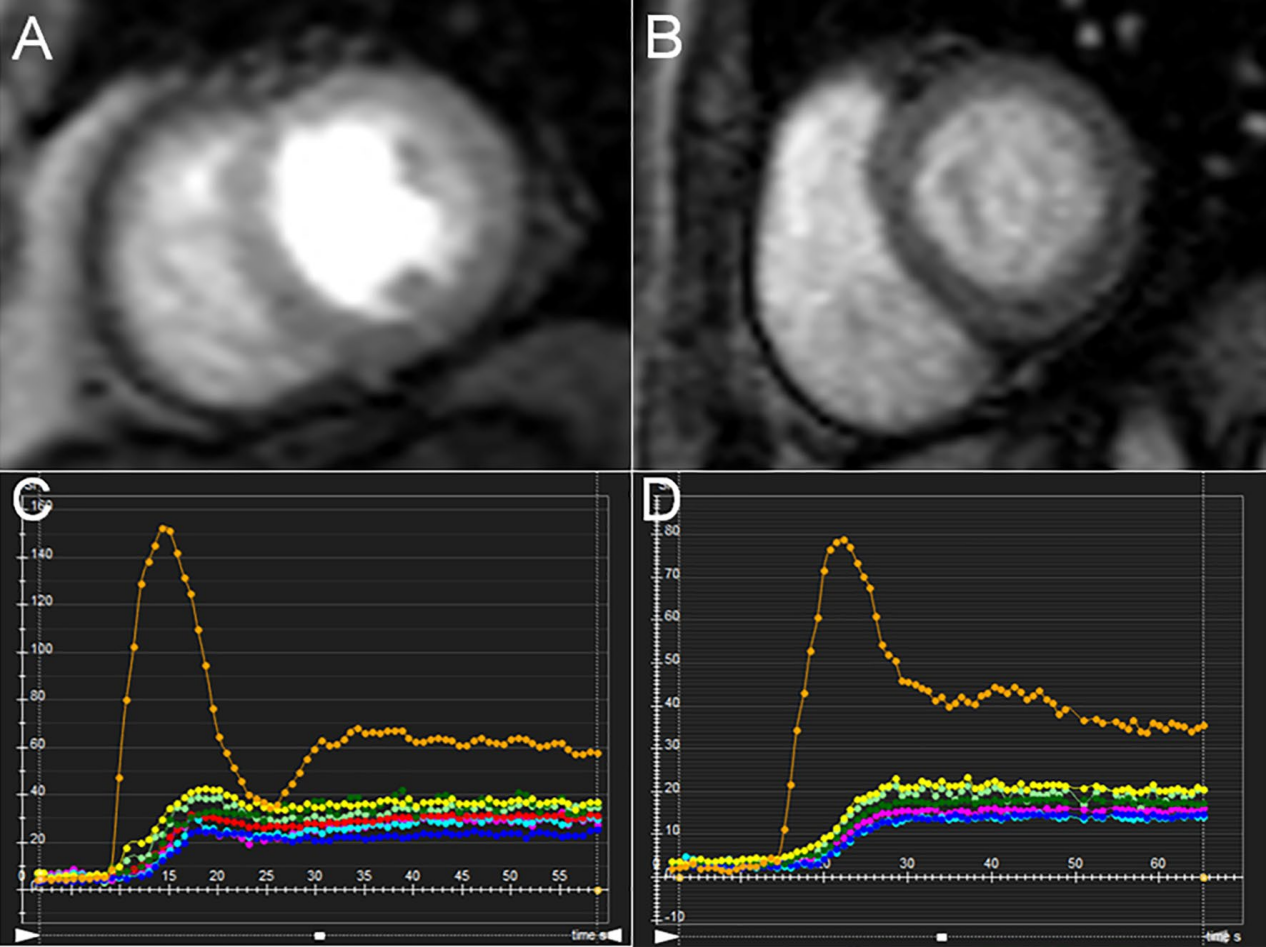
Representative first-pass myocardial perfusion MR images and signal intensity-time curves in normal controls (A, C) and patients with T2DM (B, D)
